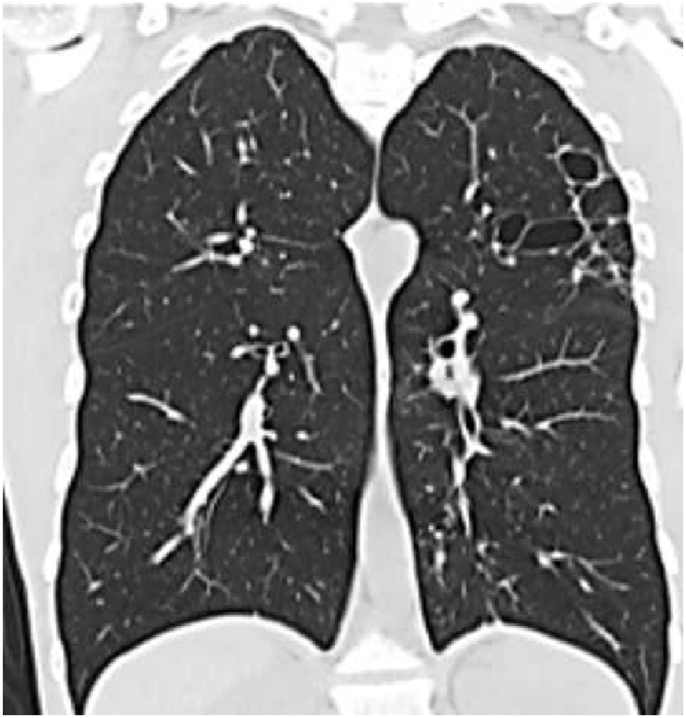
CT chest, March 2021: left upper lobe cystic changes resolved.
